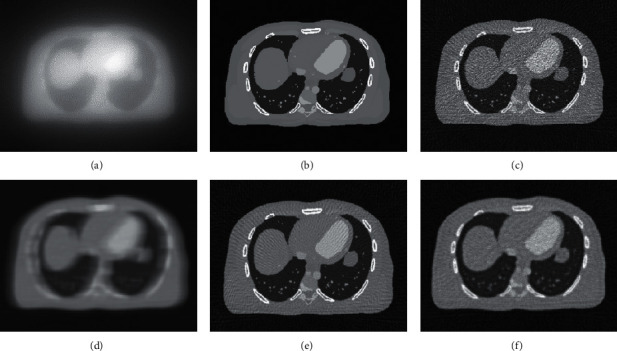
Low-dose HRCT image reconstruction: (a, d) images added with noise; (b) original image; and (e, c, f) reconstructed images.
